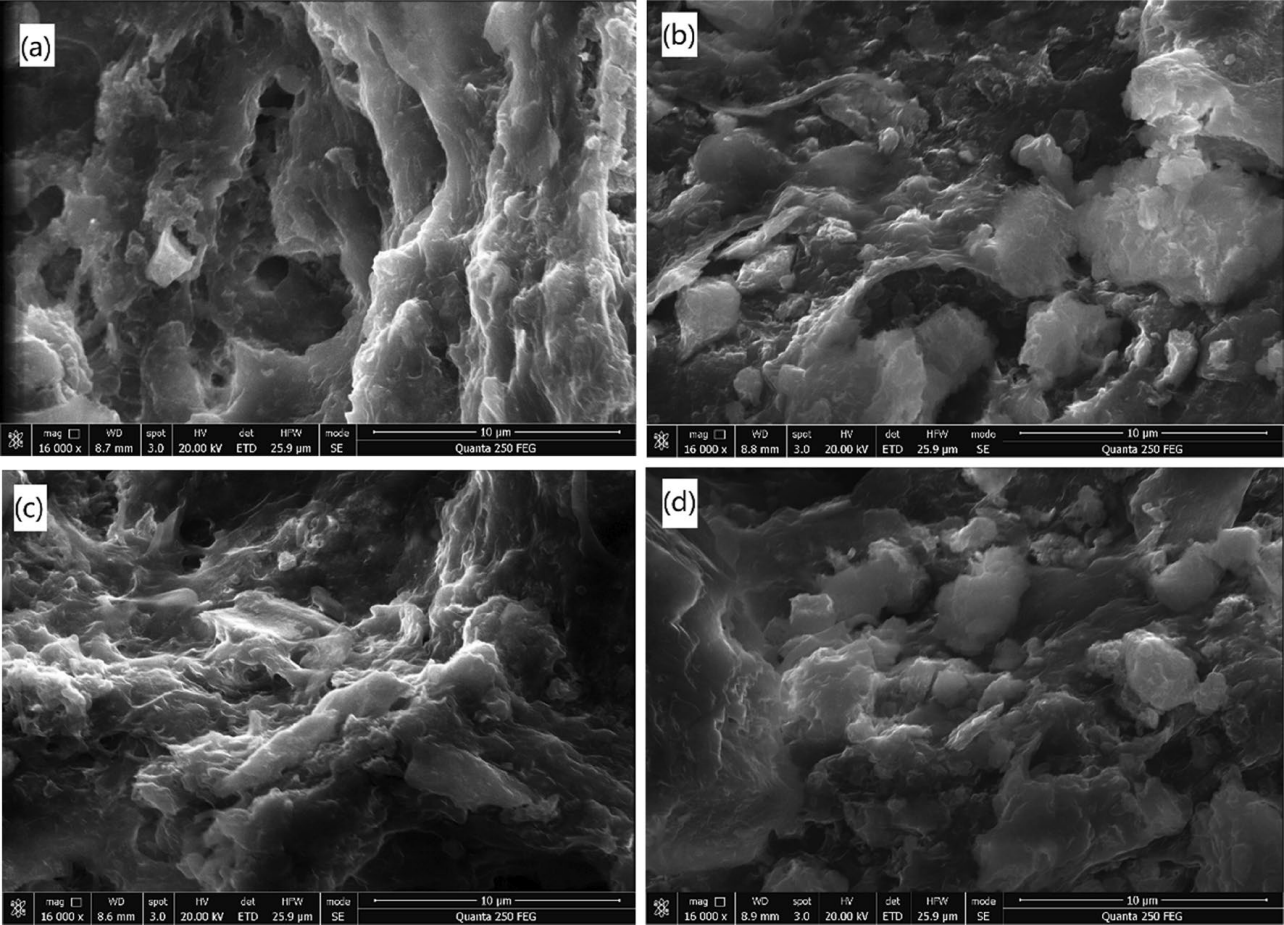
SEM images of sludge flocs: (a) raw sludge and conditioned by (b) ATP, (c) St-CTA, and (d) St-CTA in conjunction with ATP at respective optimal dose, that is, St-CTA is 16.00 mg/gTSS and ATP is 14.00 kg/m^3^.
